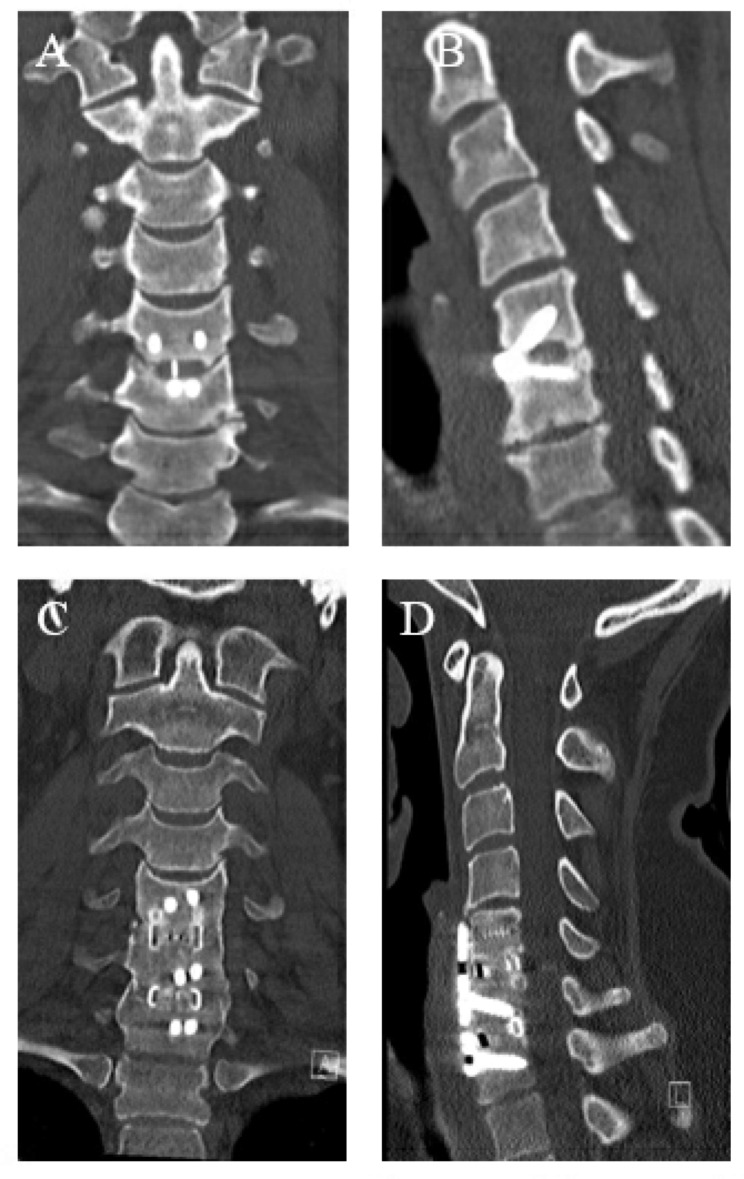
Coronal and sagittal postoperative CT 5.5 years after the index procedure shows pseudoarthrosis C5/6 (A + B). Coronal and sagittal postoperative CT after revision with satisfactory fusion (D + E)
